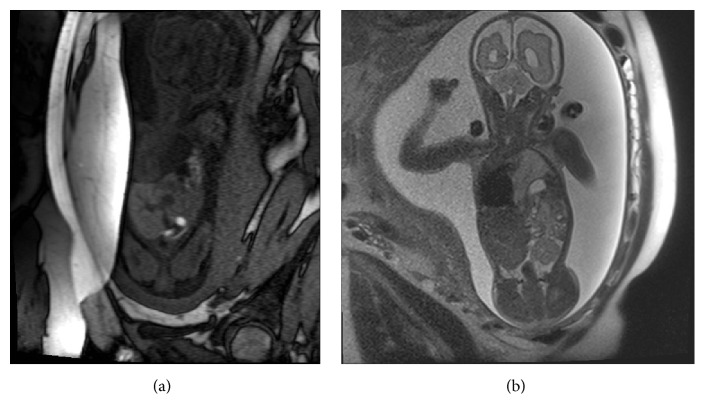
(a) T1-weighted: coronal MRI imaging at 28-week gestation showing left pulmonary bronchopulmonary foregut malformation and herniated bowel in the left hemithorax and displacement of the cardiac structures to the right side. (b) T2-weighted: coronal MRI imaging at 28-week gestation showing left pulmonary bronchopulmonary foregut malformation and herniated bowel in the left hemithorax and displacement of the cardiac structures to the right side.
